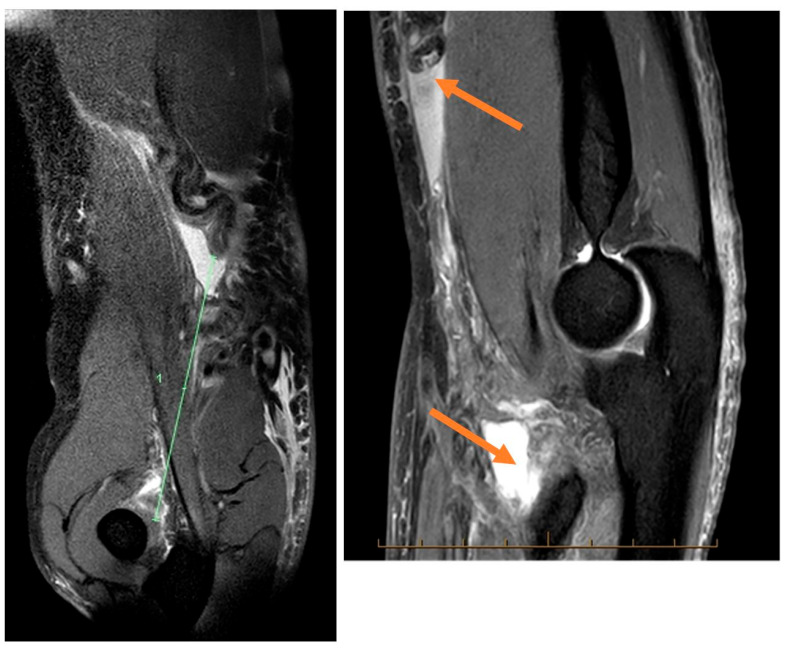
Distal biceps tendon MRI.
